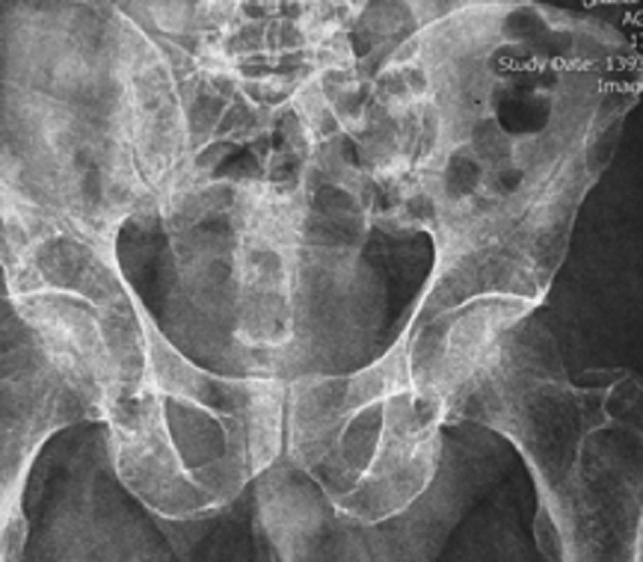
Lytic lesions in patient's hip radiography
